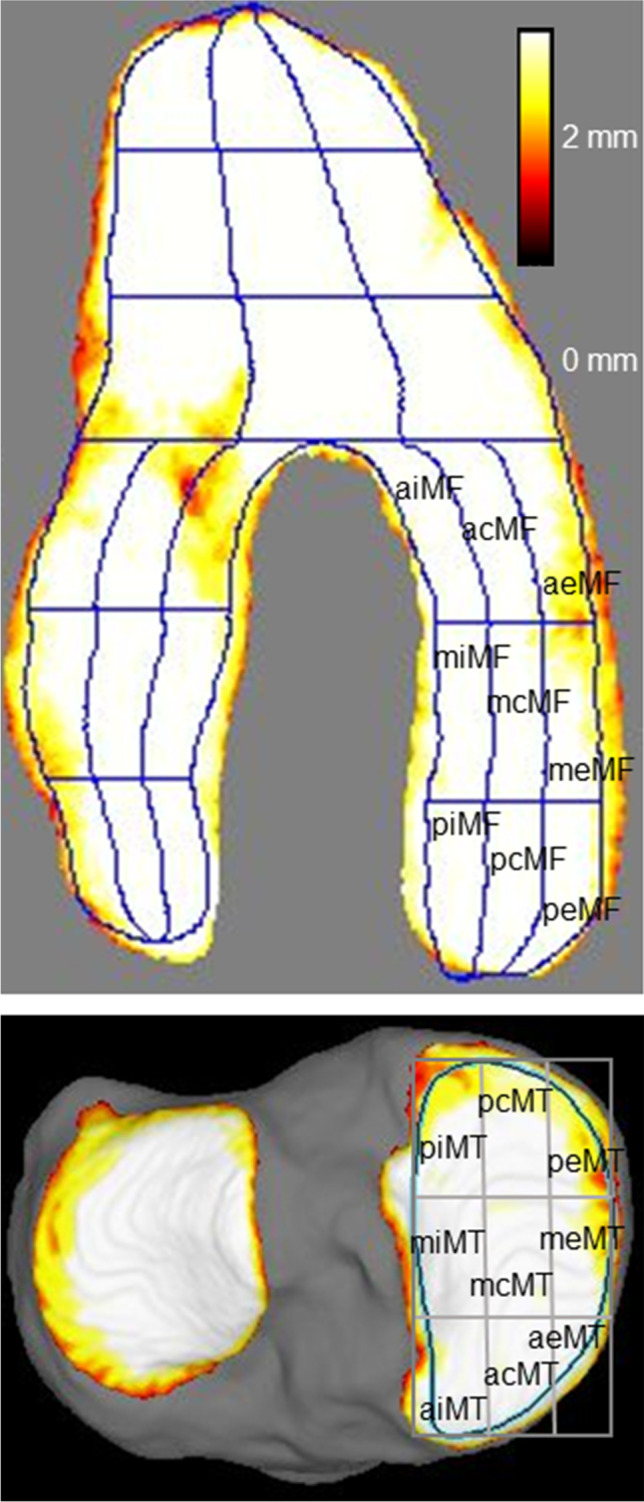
MRI cartilage thickness mapping and subregions of the knee. MF, medial femoral; aiMF, anterior internal MF; acMF, anterior central MF; aeMF, anterior external MF; miMF, middle internal MF; mcMF, middle central MF; meMF, middle external MF; piMF, posterior internal MF; pcMF, posterior central MF; and peMF, posterior external MF. MT, medial tibial; piMT, posterior internal MT; pcMT, posterior central MT; peMT, posterior external MT; miMT, middle internal MT; mcMT, middle central MT; meMT, middle external MT; aiMT, anterior internal MT; acMT, anterior central MT; and aeMT, anterior external MT
